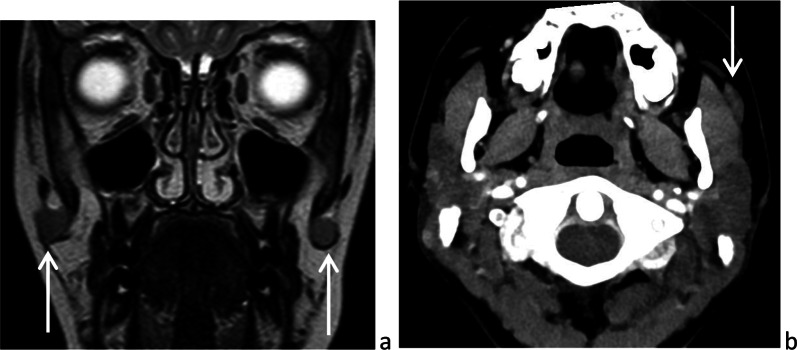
Accessory parotid glands on frontal *T*_2_-weighted image (a) and axial CT after intravenous injection of contrast medium (b)
